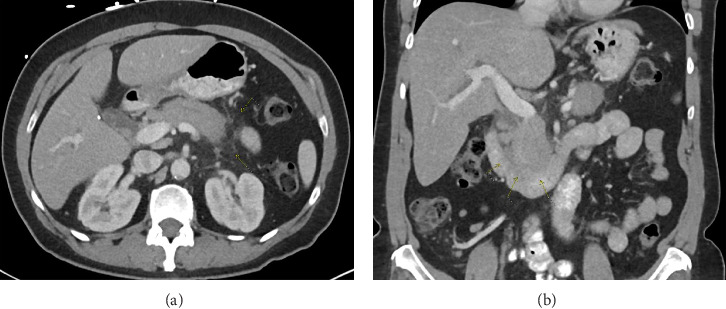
CT abdomen and pelvis. Axial images (a) suggest acute pancreatitis with mild fat stranding surrounding the pancreas, and the coronal images (b) show loss of the interface between the pancreas and the duodenum.
